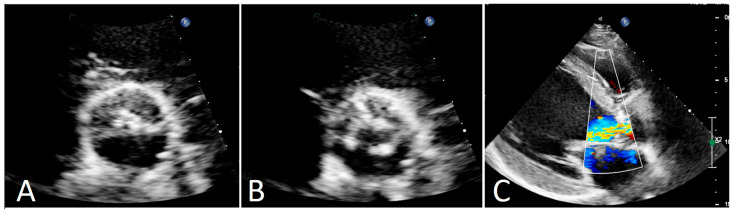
Congenital malformations of the aortic valve have different variants with various incidences in the population. Bicuspid aortic valves are the most common aortic anomaly (up to 2%), followed by unicuspid (0.02%) and then quadricuspid aortic valves (QAVs) (less than 0.01%) [1]. Historically, QAVs were encountered during open heart surgery or postmortem. Nowadays, in the majority of cases, the diagnosis is made by echocardiography (51%), intraoperatively (22.6%), and at autopsy (15.6%) [2]. To date, the literature regarding QAV characteristics is limited, with very little data describing disease presentation and treatment options. We present a rare case of surgical treatment of a patient with a QAV. A 63-year-old man complained of fatigue and moderate-to-severe dyspnea (NYHA class II) with a history of hypertension. Physical examination revealed diastolic murmur at the left-middle sternal border. Preoperative transthoracic echocardiography showed severe central aortic regurgitation as a result of malcoaptation of the presumably bicuspid aortic valve with concomitant mild-to-moderate aortic stenosis with a maximum gradient of 40 mm Hg (mean gradient of 22 mm Hg) and a valve area of 1.6 cm^2^ ((A)—systole, (B)—diastole, (C)—aortic regurgitation jet) (Figure 1). Left-ventricular dysfunction was present: end-diastolic diameter was 60 mm, ejection fraction was 40% with diffuse hypokinesis. Ascending aorta and aortic root were normal. Origins of the coronary arteries ostia were typical. Coronary angiography revealed no stenoses. The patient was scheduled to undergo elective surgery.
